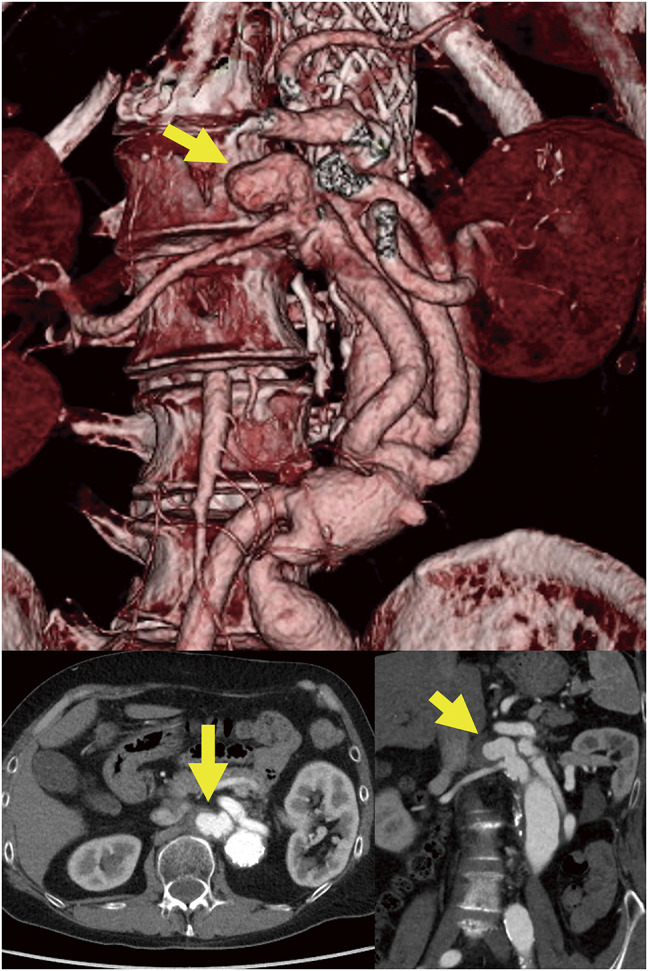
Three-dimensional cardiac computed tomography angiography revealed a right renal artery aneurysm at the prosthetic anastomosis site (arrow). The patient had previously undergone thoracoabdominal aortic repair. The diameter of the right renal artery was 6 mm, and its length was 70 mm. The renal branch of prosthetic graft was 10 mm in diameter and 95 mm in length.
